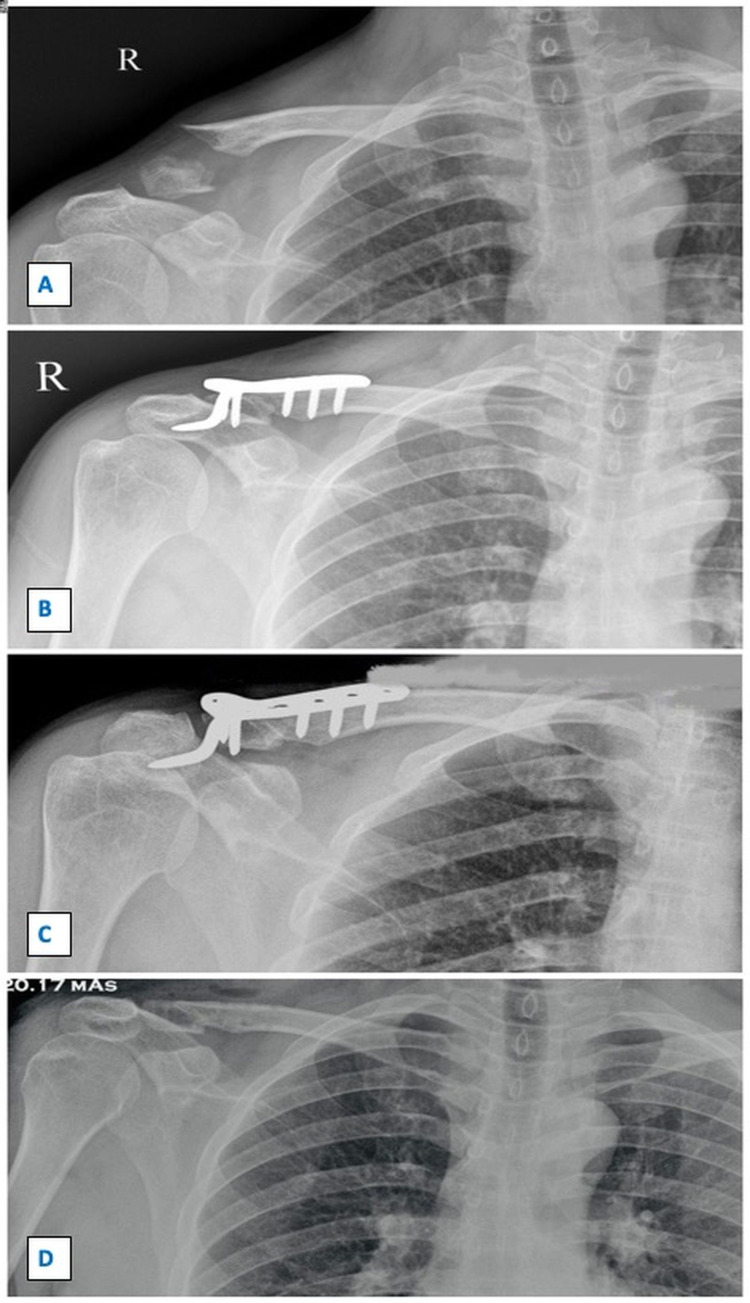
Sequential radiographs showing fracture of right lateral end clavicle managed by clavicular hook plate. Figure 6A shows the pre-operative radiograph with displaced right lateral end clavicle fracture. Figure 6B shows immediate post-operative radiograph managed by clavicular hook plate. Figure 6C shows united fracture at three-month follow-up with subacromial osteolysis and impingement. Figure 6D shows the radiograph after clavicular hook plate removal with the complete union at the fracture site.
